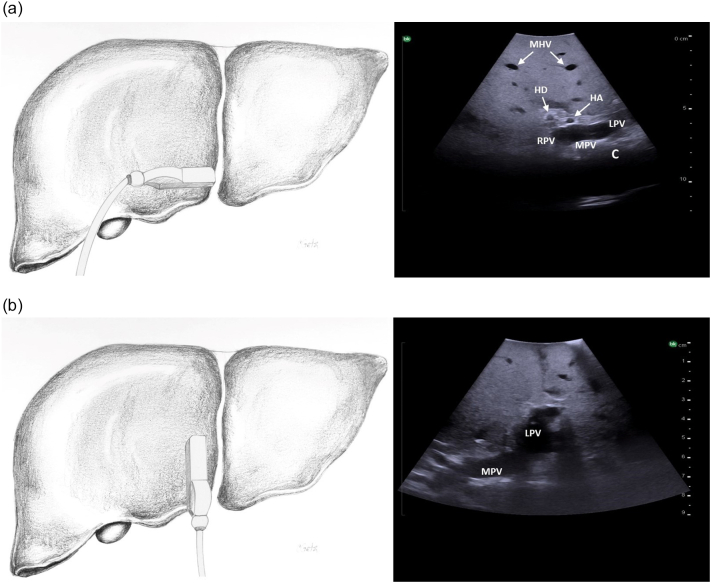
Intraoperative ultrasound of the portal vein bifurcation. a) Transverse probe placement and ultrasound image, b) Longitudinal probe placement and ultrasound image. MPV main portal vein, LPV left portal vein, RPV right portal vein, MHV middle hepatic vein tributaries, HD bile duct, HA hepatic artery, C caudate.
